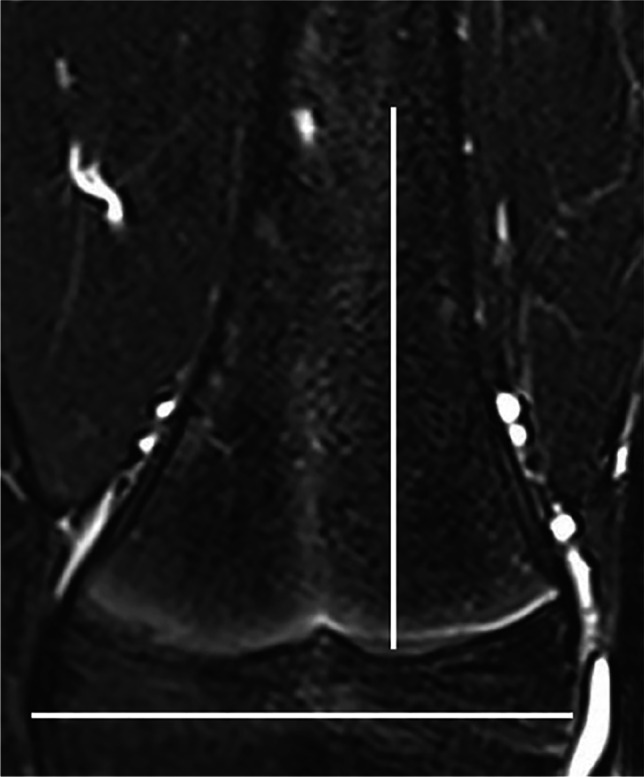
A coronal water-only Dixon T2-weighted magnetic resonance image in a 16-year-old girl with an area of increased signal intensity just within the borders of the metaphysis of the tibia, as calculated by the maximal width of the epiphysis
